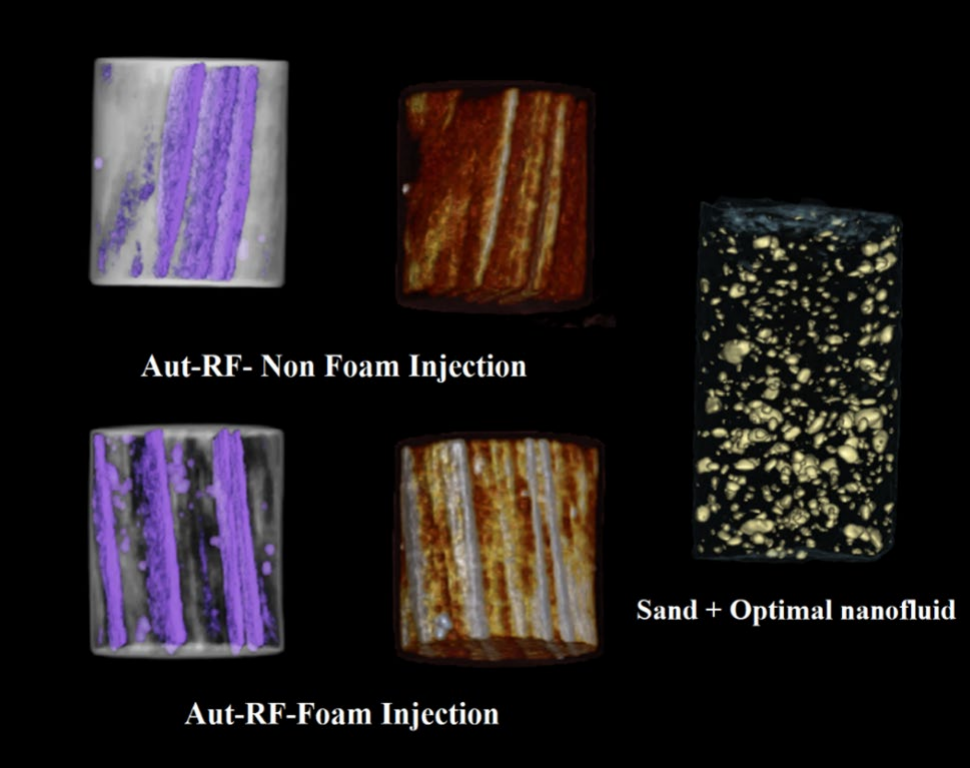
CT scan images of the injected core (liquid and foam) and cured samples (optimized sand and nanofluid).
